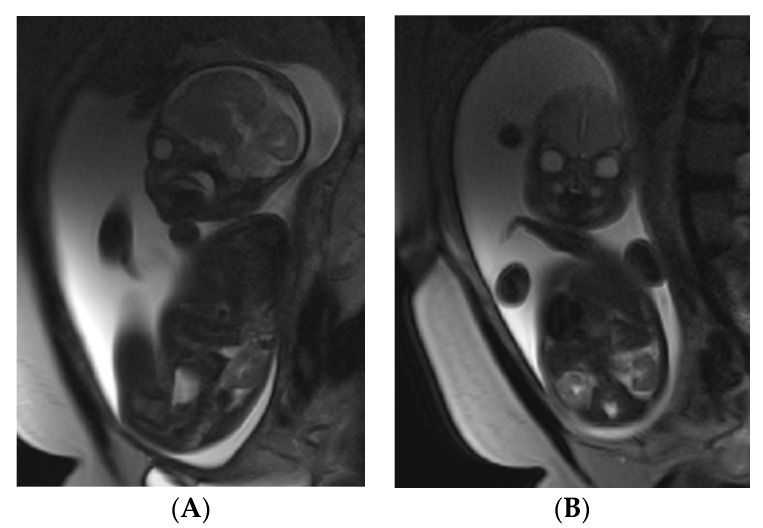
(A,B) Sagittal and coronal MRI scan of the fetus. Herniation in the left side of the diaphragm, the stomach, bowel loops and the left liver lobe in the chest cavity.
